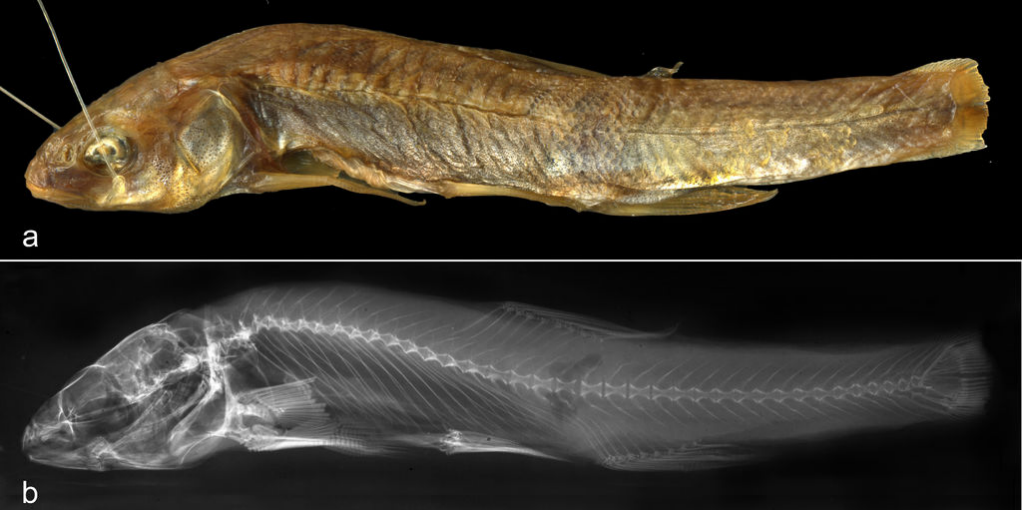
Photo (a) and x-ray (b) of the lectotype *Phoxinus
